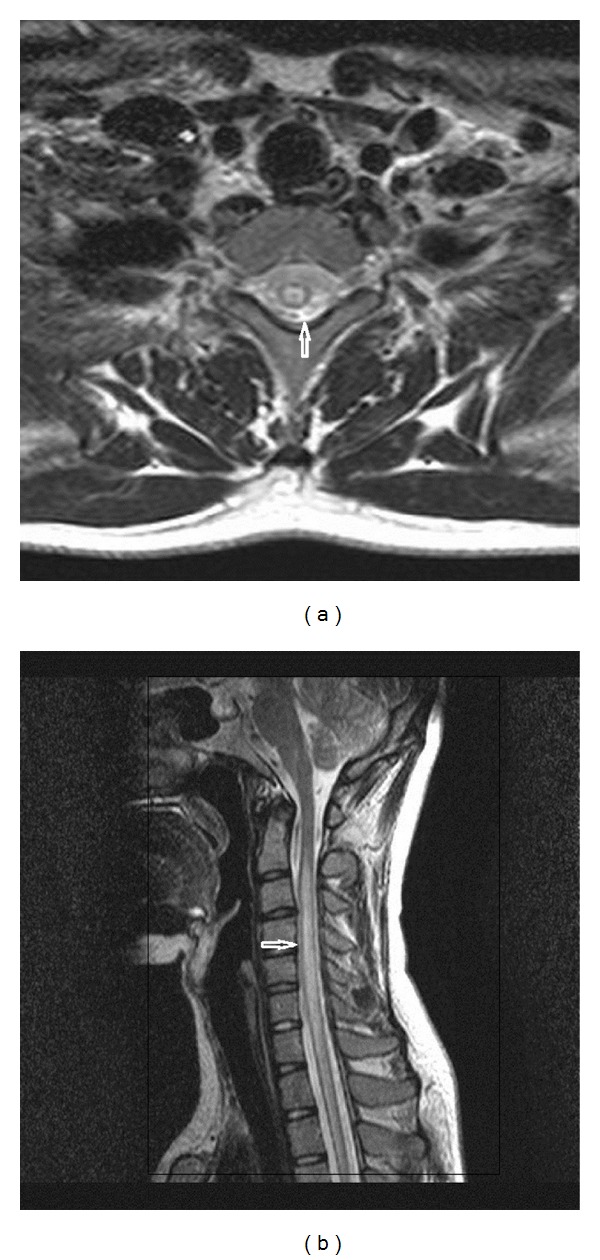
T2-weighted axial (a) and sagittal (b) MR images of the cervical spine demonstrate long segmental intramedullary high signal intensity suggesting transverse myelitis.
